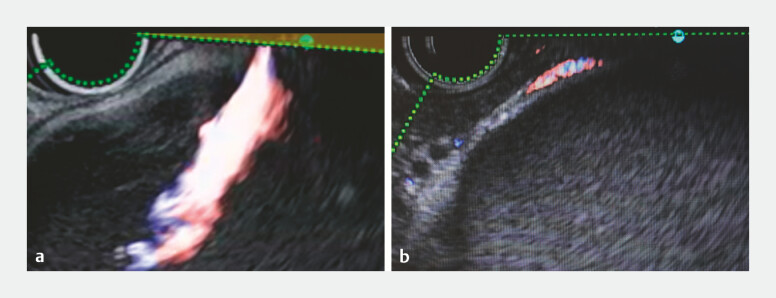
Color Doppler imaging comparison between the (
